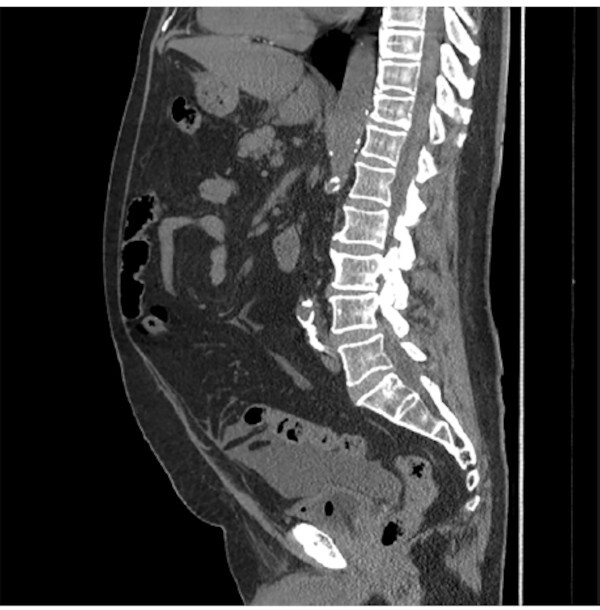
Non-contrast CT-scan with intraperitoneal fluid compatible with ascites.
